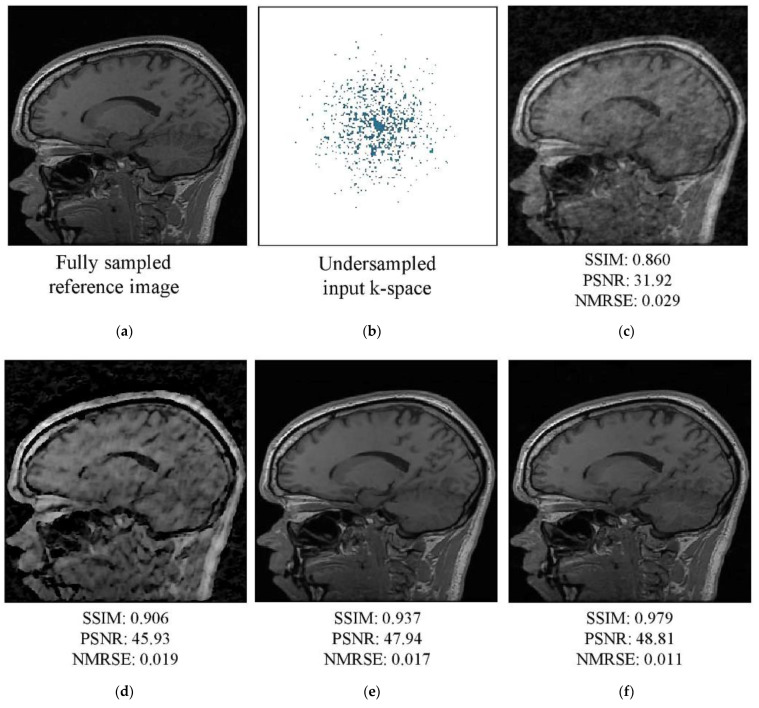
MRI images from the test dataset (slice No. 100) for acceleration factor (AF) 4: (a) fully sampled reference image and (b) undersampled k-space. Image reconstructed by the (c) inverse fast Fourier transform (IFFT), (d) Unet, (e) W-net, and (f) RA-CNN methods.
